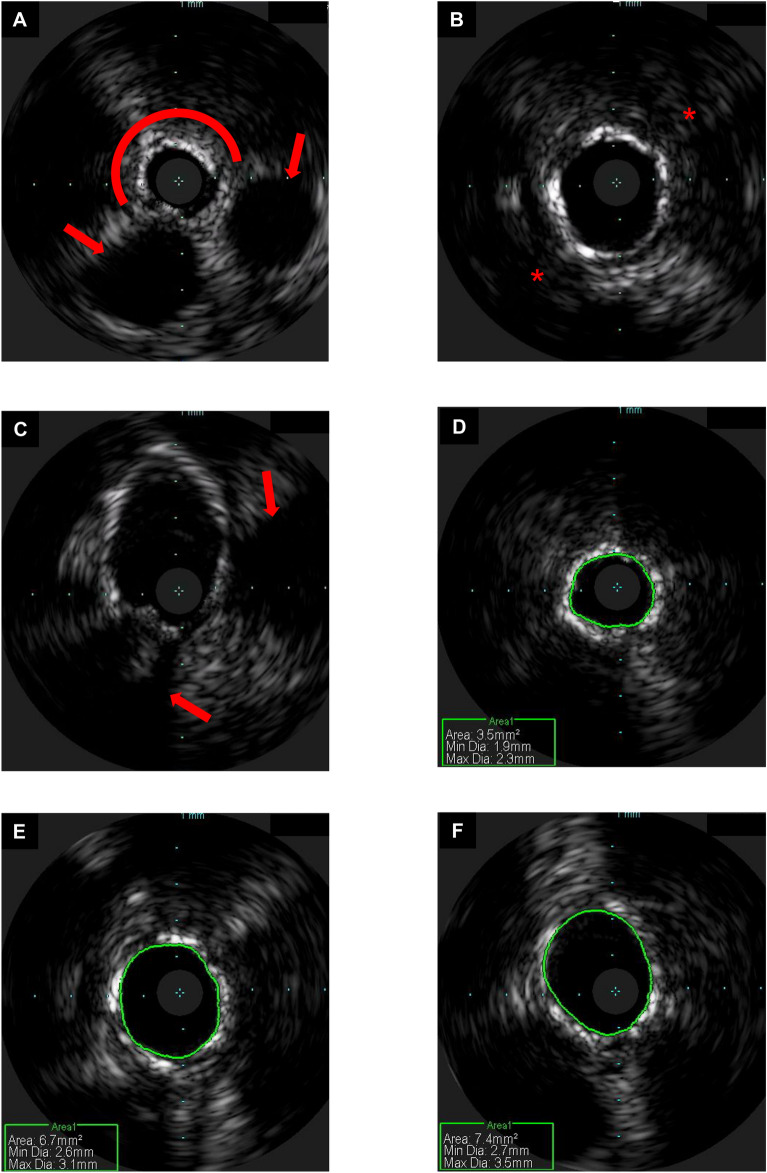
Intravascular imaging of the amputated human limb model using intravascular ultrasound. Intravascular ultrasound study of the amputated human limb model performed using the Eagle Eye Platinum catheter (Volcano, Philips, Amsterdam, Netherlands). (A) Still frame of the distal posterior tibial artery, demonstrating superficial calcification (7 o’clock to 3 o’clock, highlighted by red arc) without significant luminal obstructive disease. There are surrounding extraluminal vessels (highlighted by red arrows), which likely represent posterior tibial venous branches veins running alongside the posterior tibial artery. (B) Still frame of the mid posterior tibial artery, demonstrating concentric calcification, with loss of signal penetration beyond the calcium deposits (highlighted by red *). (C) Still frame demonstrating the tibioperoneal bifurcation, as the posterior tibial artery joins the peroneal artery (highlighted by red arrows). (D–F) Minimal lumen area measurements in the distal (D), mid (E) and proximal (F) posterior tibial artery. The interval between crosshair indicators represents 1 mm.
